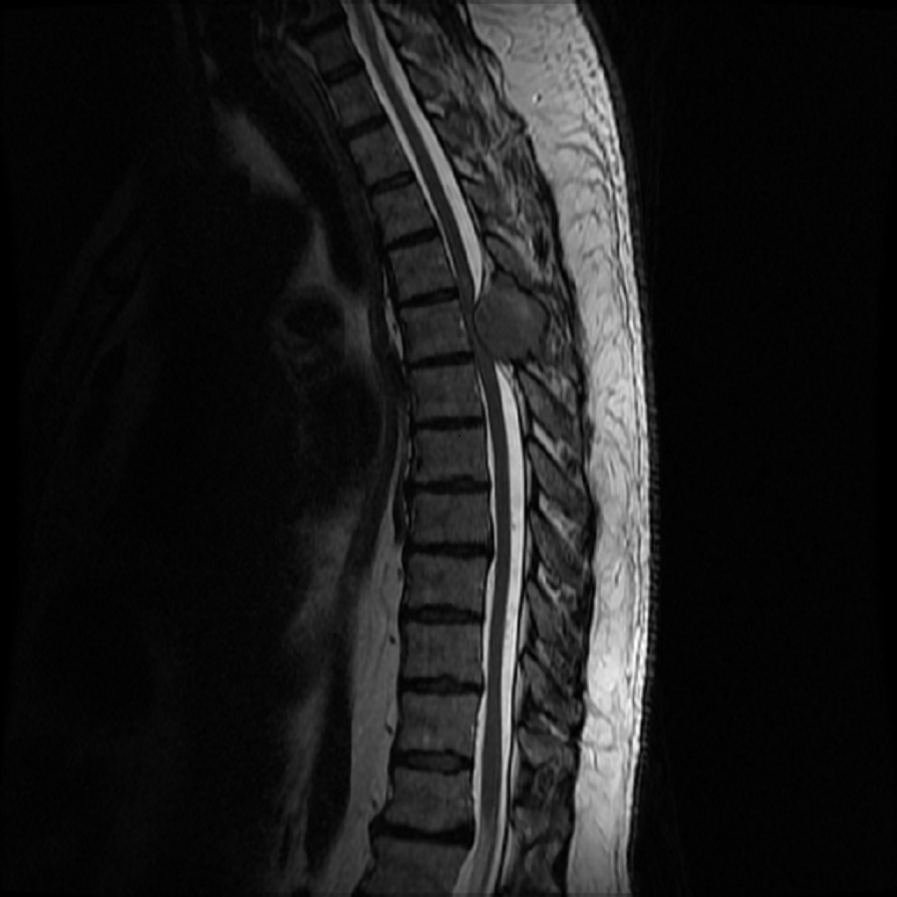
Preoperative T2W sagittal images. MRI of the thoracic spine T2W sagittal images revealed an approximately 2.9 x 3.8 x 3.7 cm extradural midline mass centered in the posterior elements of T5 from T3-T4 to T6-T7. The mass extended into the spinal canal with severe thoracic cord compression. There was increased signal within the spinal cord from the lower T4 level to the upper T6 level consistent with edema. No extension into the neural foramina was noted.
